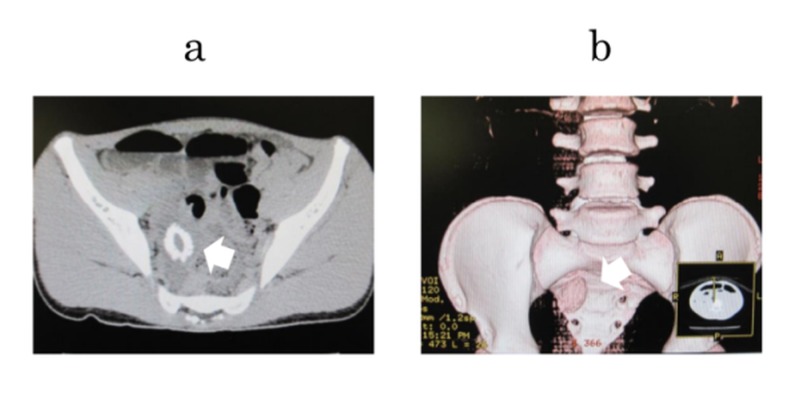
Abdominal CT A foreign body was detected in the small intestine (arrow). The shape of the object was oval. a: Coronal plain abdominal CT. b: Three-dimensional construction from the CT images. CT: computed tomography
